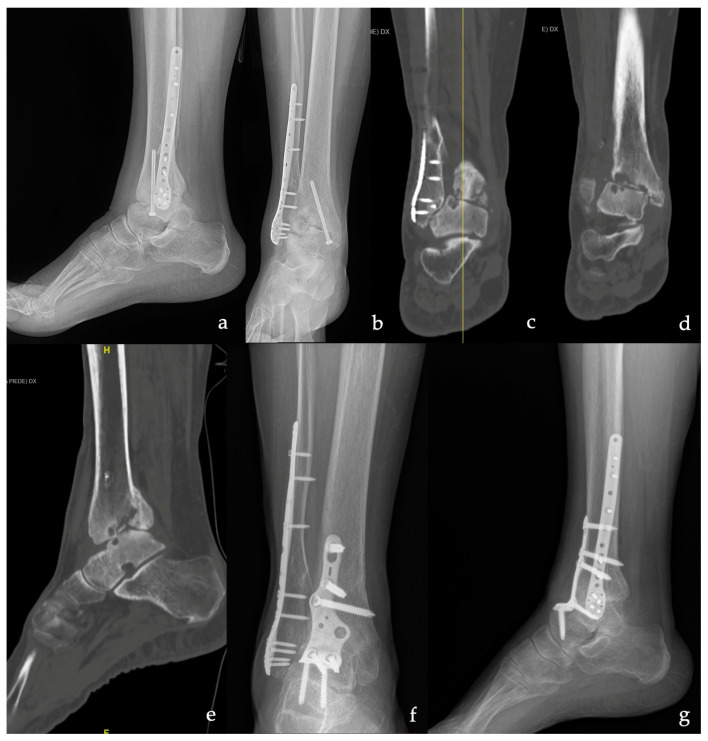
A 74-year-old woman with secondary post-traumatic TT osteoarthritis (OA). (a,b) Pre-operative X-ray in the lateral and anteroposterior view. (c,d) Coronal pre-operative CT scan showing severe OA of the TT joint. (e) Sagittal pre-operative CT scan at the level of the yellow line in figure (c). (f,g) Anteroposterior and lateral X-ray at 6 months of follow-up from tibiotalar (TTC) arthrodesis with the anterior plate showing complete bone union.
